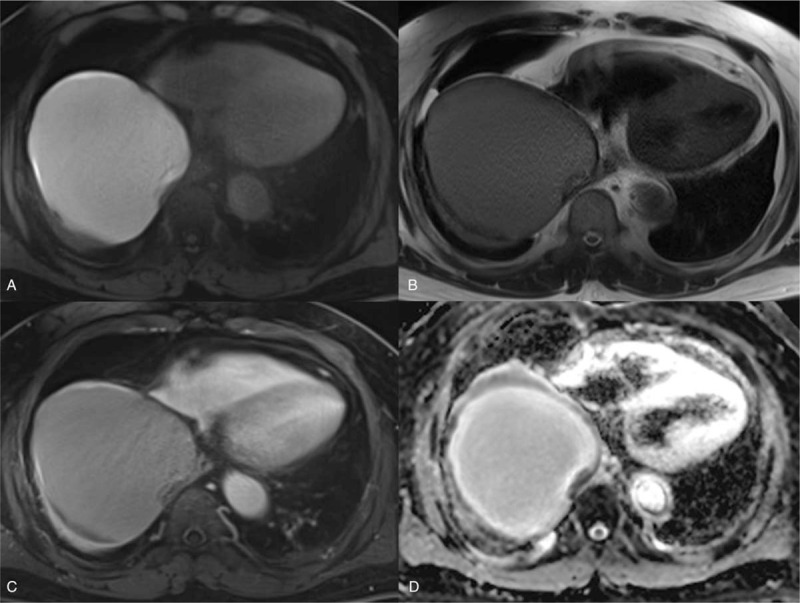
(A–D) T1-weighted thoracic MRI, T2-weighted thoracic MRI, T1-weighted enhanced thoracic MRI, and ADC map. The huge mass shows higher signal intensity than CSF on T1-weighted images (A) and an intermediate-to-low signal intensity compared to CSF on T2-weighted images (B), suggestive of a cystic lesion with subacute stage hemorrhage. T1-weighted enhanced MRI (C) reveals mild irregular rim enhancement of the cystic wall with blood clots. The ADC map (D) shows high signal intensity, suggesting the cystic nature of the mass. ADC = apparent diffusion coefficient, CSF = cerebrospinal fluid, MRI = magnetic resonance image.
